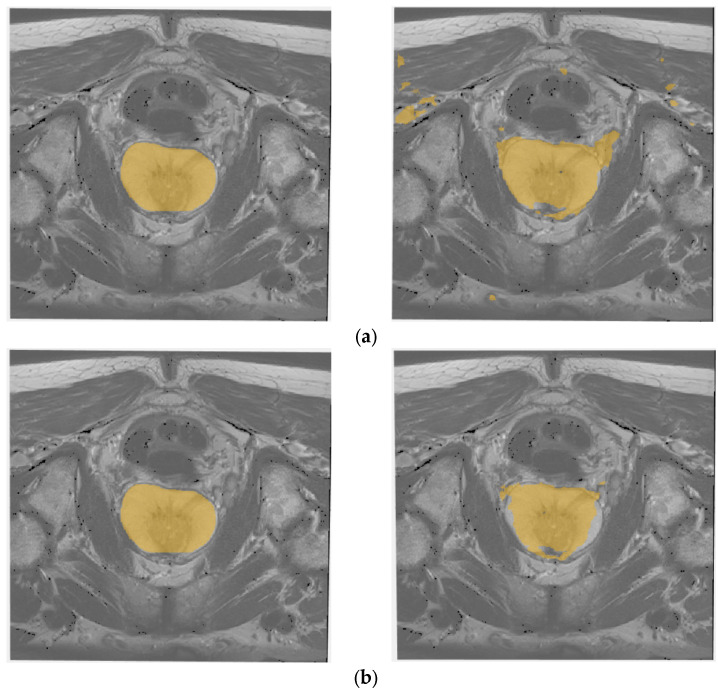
Visual comparison of prostate gland detection based on: (a) 3D U-Net (3), and (b) 3D AID-U-Net (2, 1). The slice images shown in the left column are the original CT-Scan slices accompanying with the pixel-wise annotations for prostate gland.
